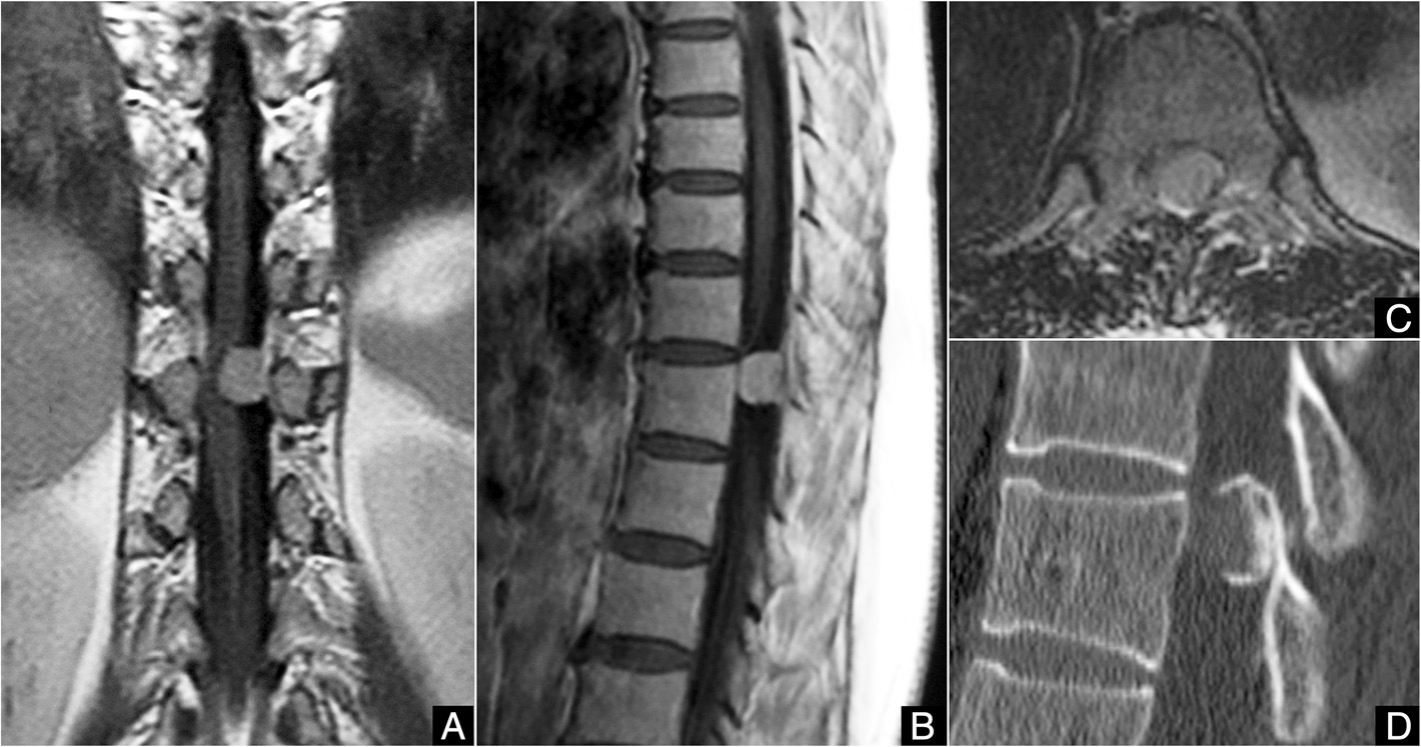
Preoperative gadolinium-enhanced T1-weighted magnetic resonance imaging (MRI) and computed tomography (CT) of the thoracic spine. a MRI in the coronal orientation, b MRI in the sagittal orientation, and c MRI in the axial orientation showing an intradural extramedullary tumor with dural tail sign located on the left and dorsal sides at the T10-T11 level, compressing the spinal cord severely. d Sagittal reconstruction CT shows extensive peripheral calcification of the tumor
